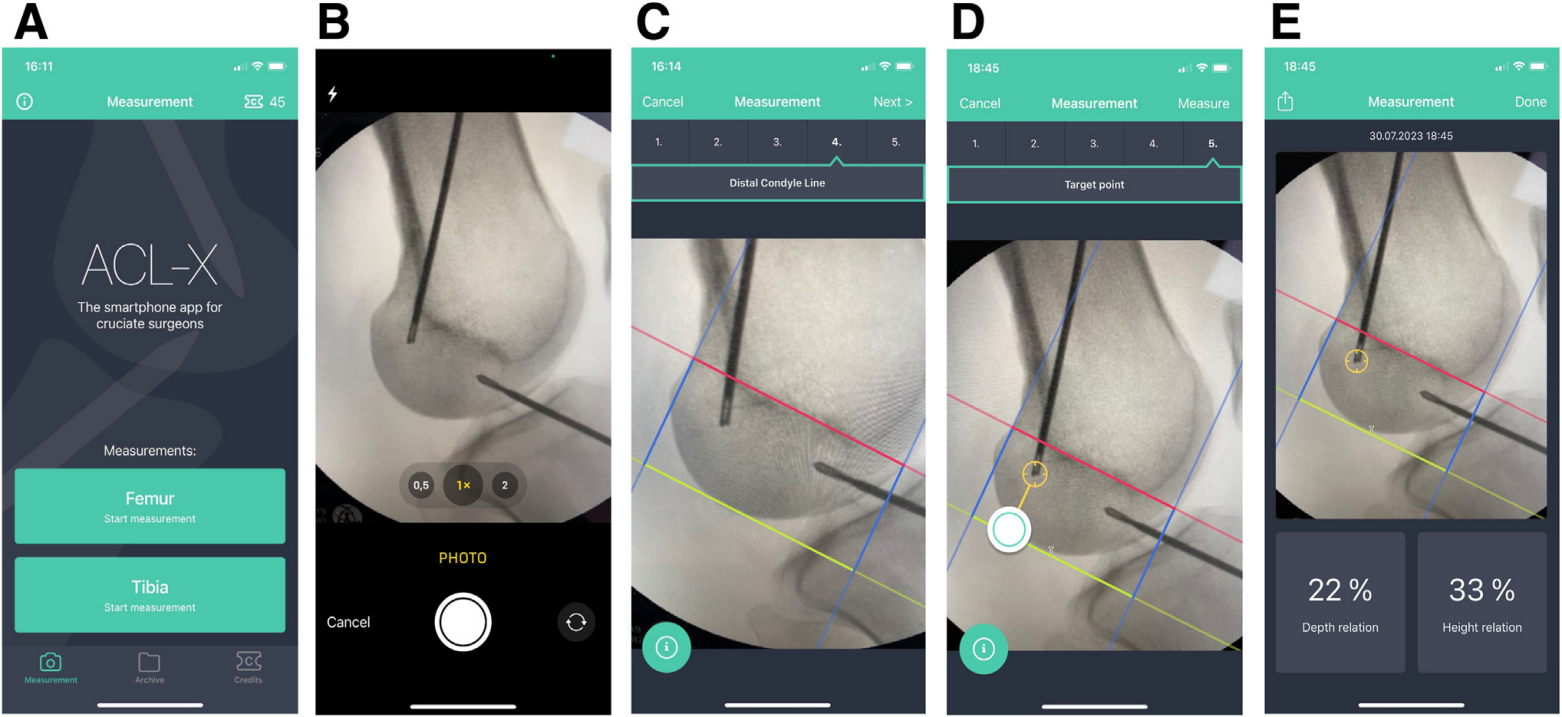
Steps for digital analysis of a true lateral radiograph using the ACL-X app. To perform this analysis, the back end of the guide pin has to be forwarded to the femoral tunnel entrance. (A) Title screen of the ACL-X app (download from Apple App Store: https://Apps.apple.com/de/app/acl-x/id1439731734, version 1.0.2 v. 21.05.2020). (B) Step 1: Open ACL-X app on your iPhone: Take a picture of a true lateral radiograph from the fluoroscopic monitor or from a computer screen and confirm the picture (you can retake the picture if it is not suitable). (C) Steps 2-5: Establish a line on the screen (in red) by touching the smartphone screen with 2 fingers (ideally index and middle finger) and move and turn the line so that it perfectly superimposes Blumensaat’s line. Confirm this position by pushing “next.” By touching the screen with 1 finger (usually index finger), a second line on the screen (in blue) is established. The line will be perpendicular to Blumensaat’s line. Move the line with your finger so that in crosses the endpoint of the red line at the most posterior edge of the femoral condyle. The line is confirmed by pushing “next.” By touching the screen with 1 finger again, another line (in blue) is established on the screen (also perpendicular to the red line). Move the line with your finger so that it crosses the endpoint of the red line at the most anterior edge of the femur. Confirm the line by moving to the next step. By touching the screen with 1 finger, another line (in green) is established on the screen (parallel to the red line). Move the line with your finger so that it runs as a tangent to the most distal part of the femoral condyle. Confirm the position by moving to the next step. (D) Step 6: By touching the screen with 1 finger, a bullet point appears on the screen. Move the target so that its center (bull’s eye) perfectly superimposes the back end of the guide pin on the underlying image. (E) Step 7: The app will automatically calculate the relative distance of the center of the target (bull’s eye) from the posterior condyle line (depth relation) and from the Blumensaat’s line (height relation) in percentages from the total grid’s depth and height. Both numbers are displayed on the screen. This image will be automatically saved in the archive of the app and can be retrieved any time later. In addition, it may be uploaded to another destination (such as patient’s file for documentation or surgeon’s file for personal use).
